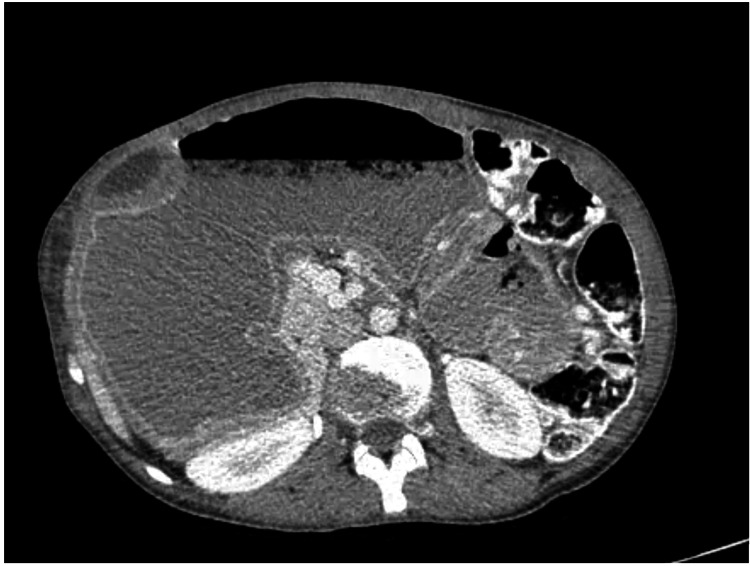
CT scan showed dilation of the stomach and associated compression.
